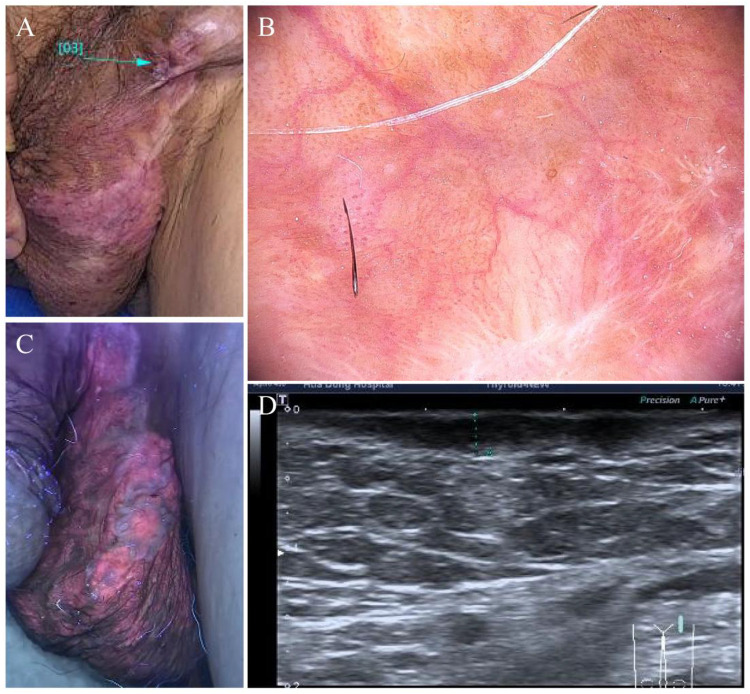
Multimodal imaging of residual EMPD before treatment. (A) Clinical image of the scrotal lesion; the green box indicates the area examined by dermoscopy. (B) Dermoscopy showing a polymorphous vascular pattern (including glomerular vessels) and milky-red areas against a yellowish background. (C) Intense red fluorescence under Wood's lamp examination 2 hours after intralesional ALA injection, confirming homogeneous and deep PpIX accumulation within the lesion, thereby validating successful drug delivery to the full depth of the tumor. (D) High-frequency ultrasound (B-mode, 20 MHz) revealing a heterogeneous, hypoechoic lesion extending into the mid-dermis, with a thickness of approximately 2.7 mm. All images have been anonymized and no unique patient identifiers are visible.
